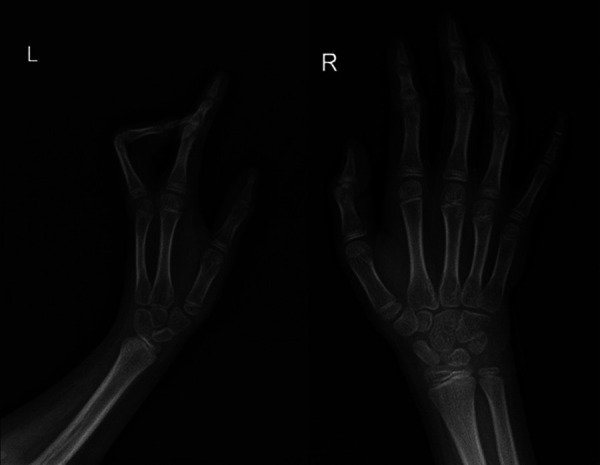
x-ray showing the absence of the left ulna, 4th and 5th metacarpals, and phalanx of the left hand.
